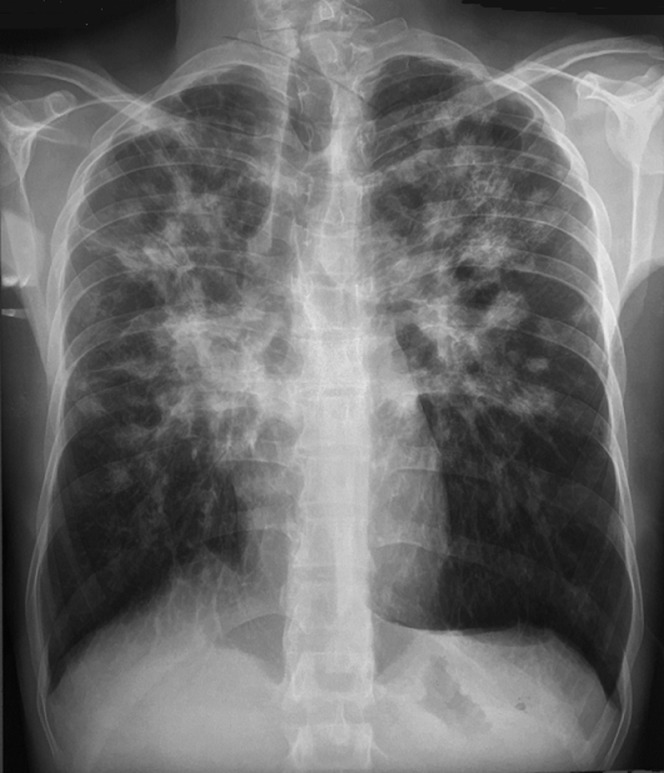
radiographie thoracique de face montrant des opacités alvéolaires et nodulaires confluentes prédominant au niveau des régions supérieures des deux champs pulmonaires
